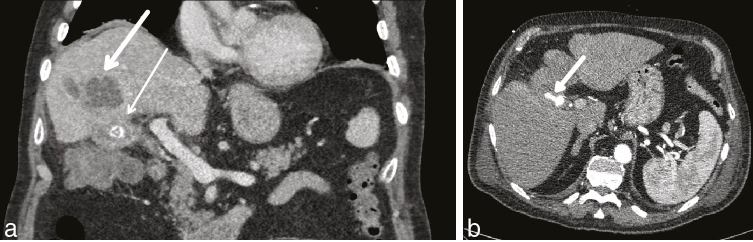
Portal venous phase CT scan. (a) Thick white arrow points to liver abscess; thin white arrow points to high density within the gallbladder. (b) Arterial phase 1 week later (arrow denotes the pseudoaneurysm).
